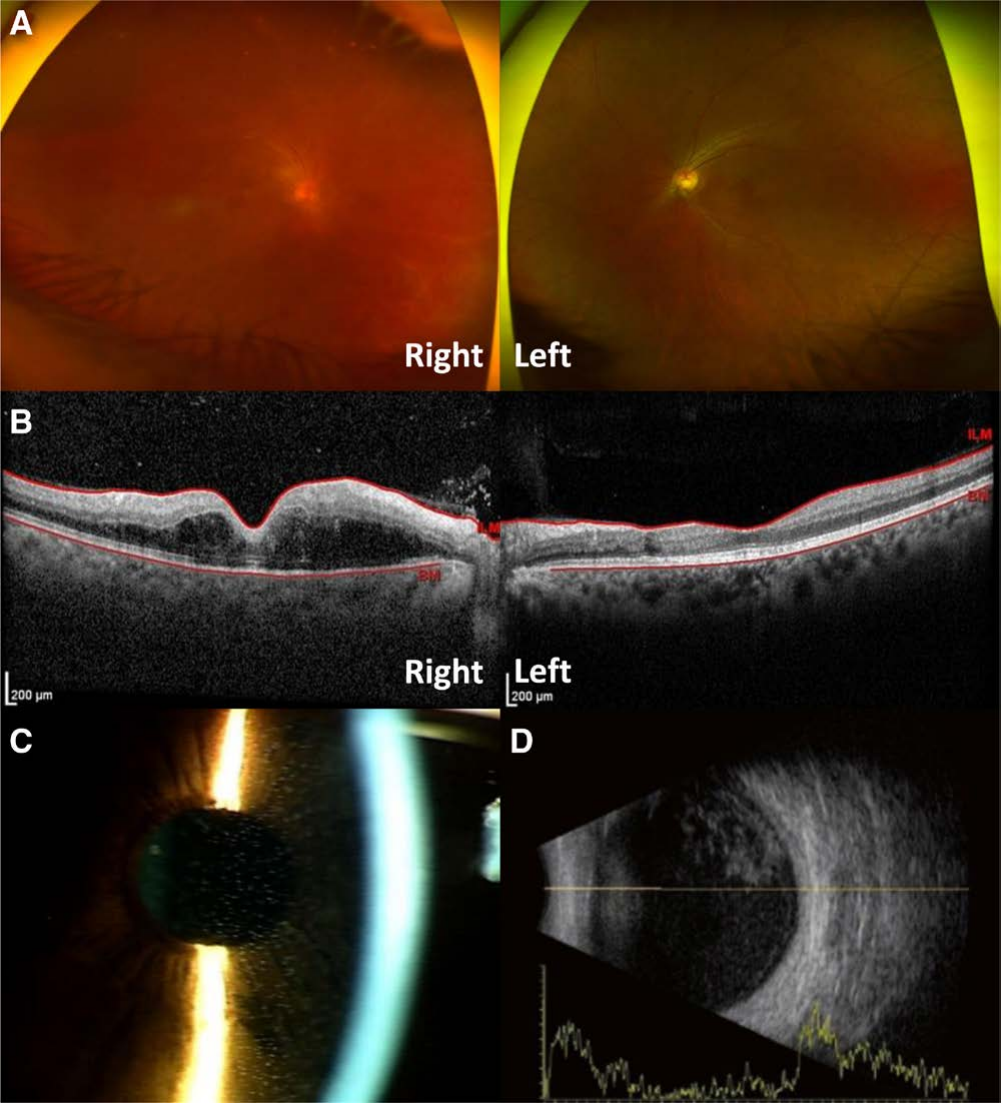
Fundus examination, optical coherence tomography, and B-scan ultrasonography the first day of a patient`s visit for decreased vision in the right eye. (A) Fundus examination reveals severe vitritis and multiple white-yellowish plaques with hemorrhages and exudation in the posterior pole of the right eye. (B) Outer retinal edema is observed on optical coherence tomography of the right eye. (C) Examination of the anterior segment reveals severe inflammation. (D) B-scan ultrasonography confirms vitreous opacification.
